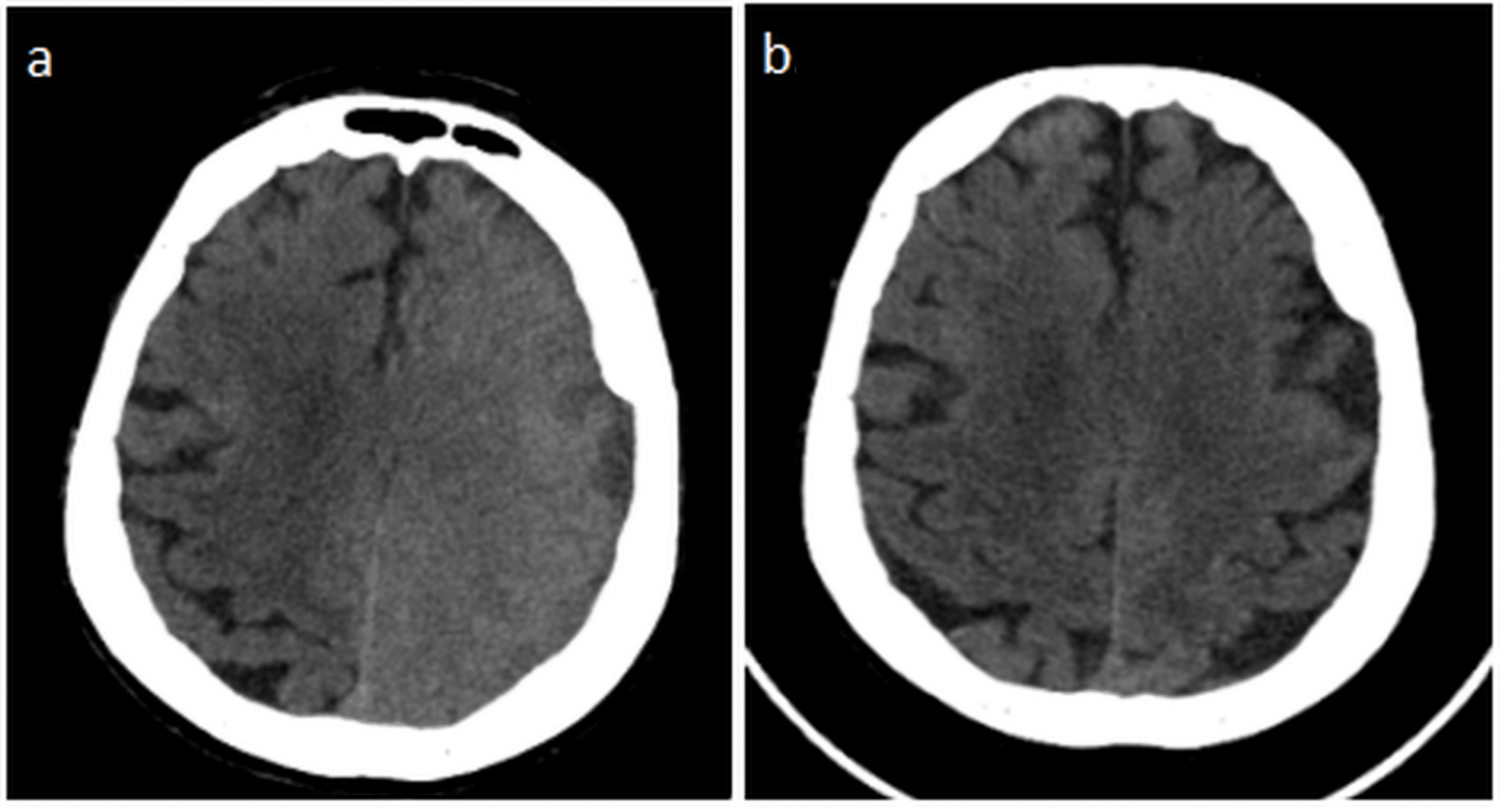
(a) Early post-procedure non-contrast brain CT demonstrating left hemispheric cortical edema and loss of gray–white matter differentiation, with no hemorrhage or mass effect, compatible with contrast-induced encephalopathy. (b) Follow-up CT after supportive therapy showing complete resolution of edema and normalization of cortical appearance
